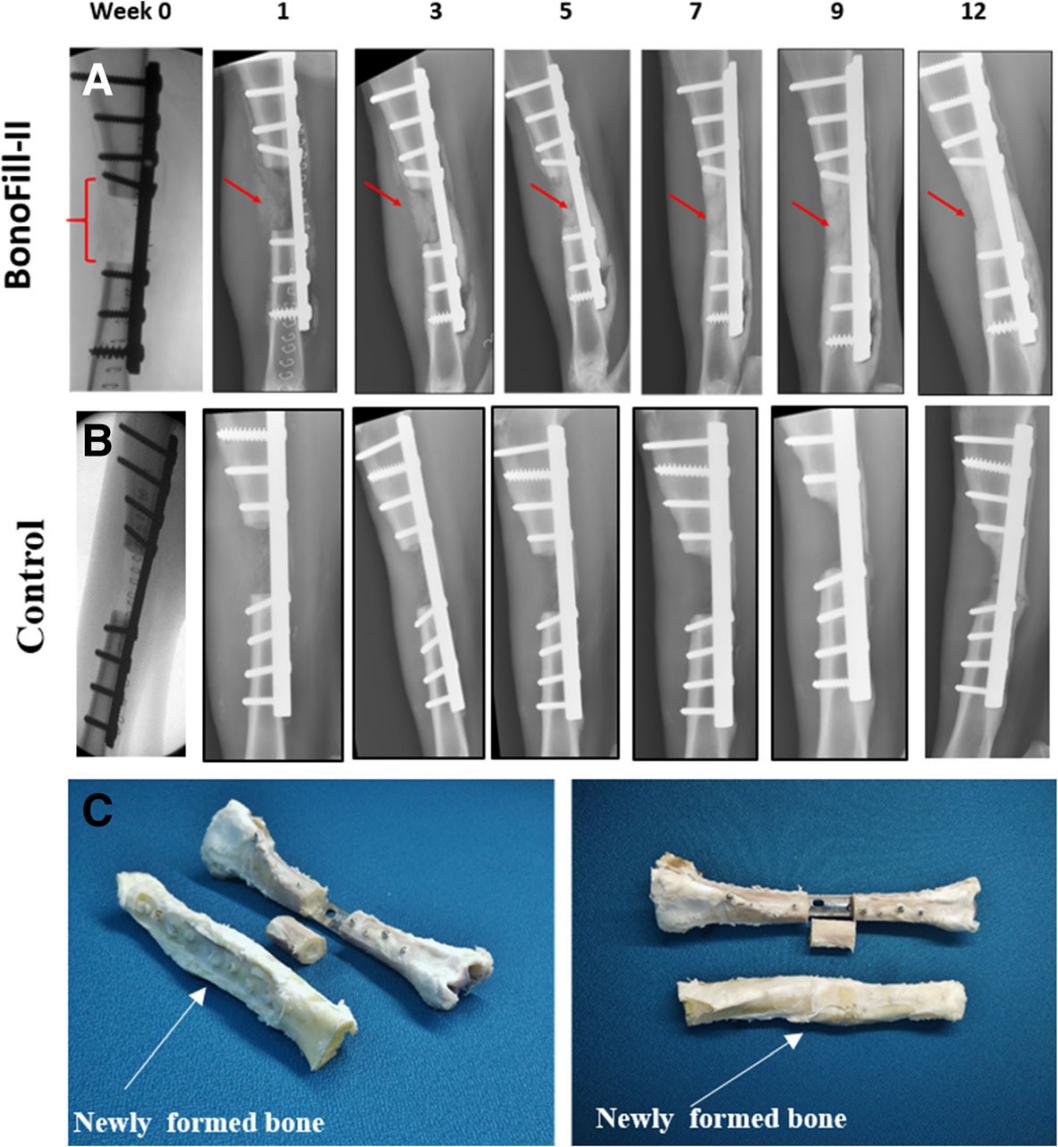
X-ray images and photographs of the treated tibia. a X-ray images of BonoFill-II-treated tibia. b Vehicle-treated tibia (control). Arrows indicate build-up of bone tissue in the gap. c Newly formed BonoFill-II-transplanted bones (front bones) after tibia extraction and models representing the 3.2-cm gap (rear bones)
